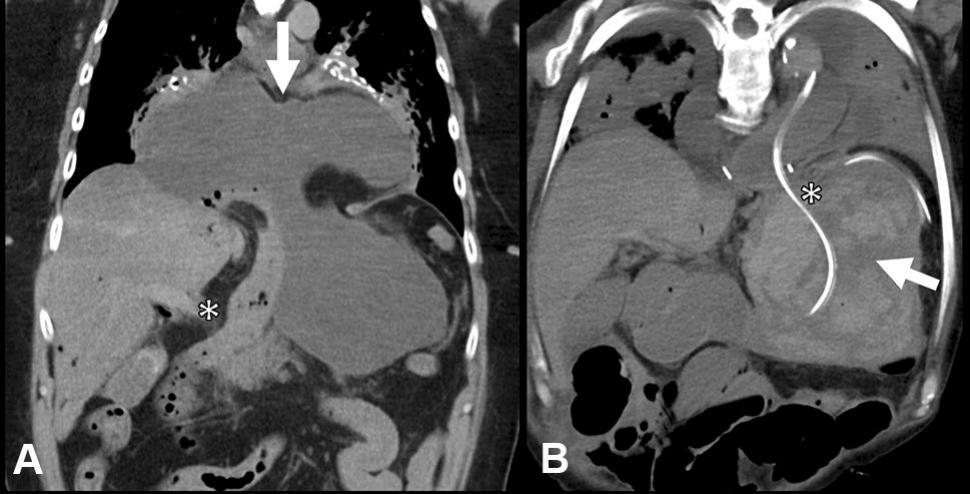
Post-mortem CT findings in gastric volvulus: case 3 (A) and case 4 (B). Coronal multiplanar reconstruction showing a distended fluid-filled stomach in a hiatus hernia (arrow) with “waisted” mid-gastric body as it passes through the hiatus and extrinsic compression to the pylorus. The proximal duodenum is collapsed (asterisk). Coronal multiplanar reconstruction showing the gastric lumen filled with mixed-density hematoma (arrow). There is no volvulus, due to reduction with a nasogastric tube (asterisk)
